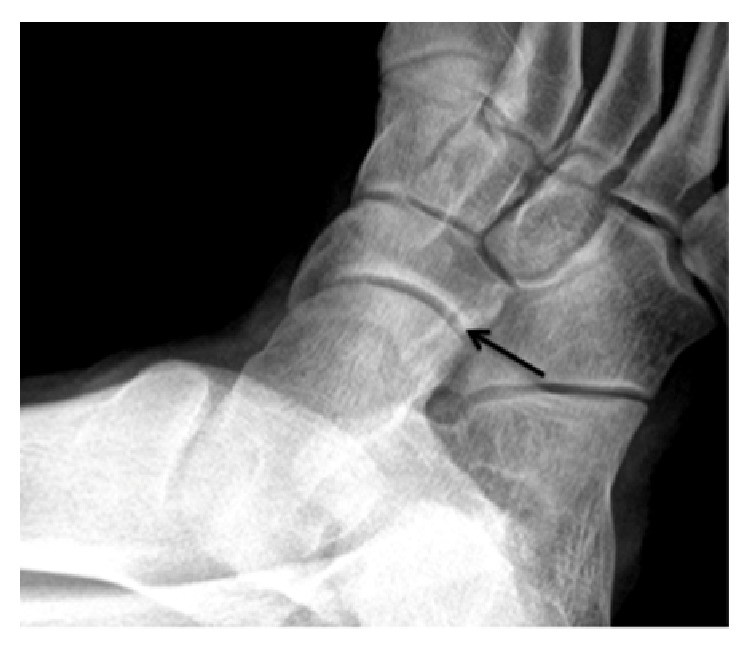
Magnified oblique radiograph of the right foot in a 17-year-old male better delineates the abnormal articulation between the cuboid (arrow) and the navicular.
